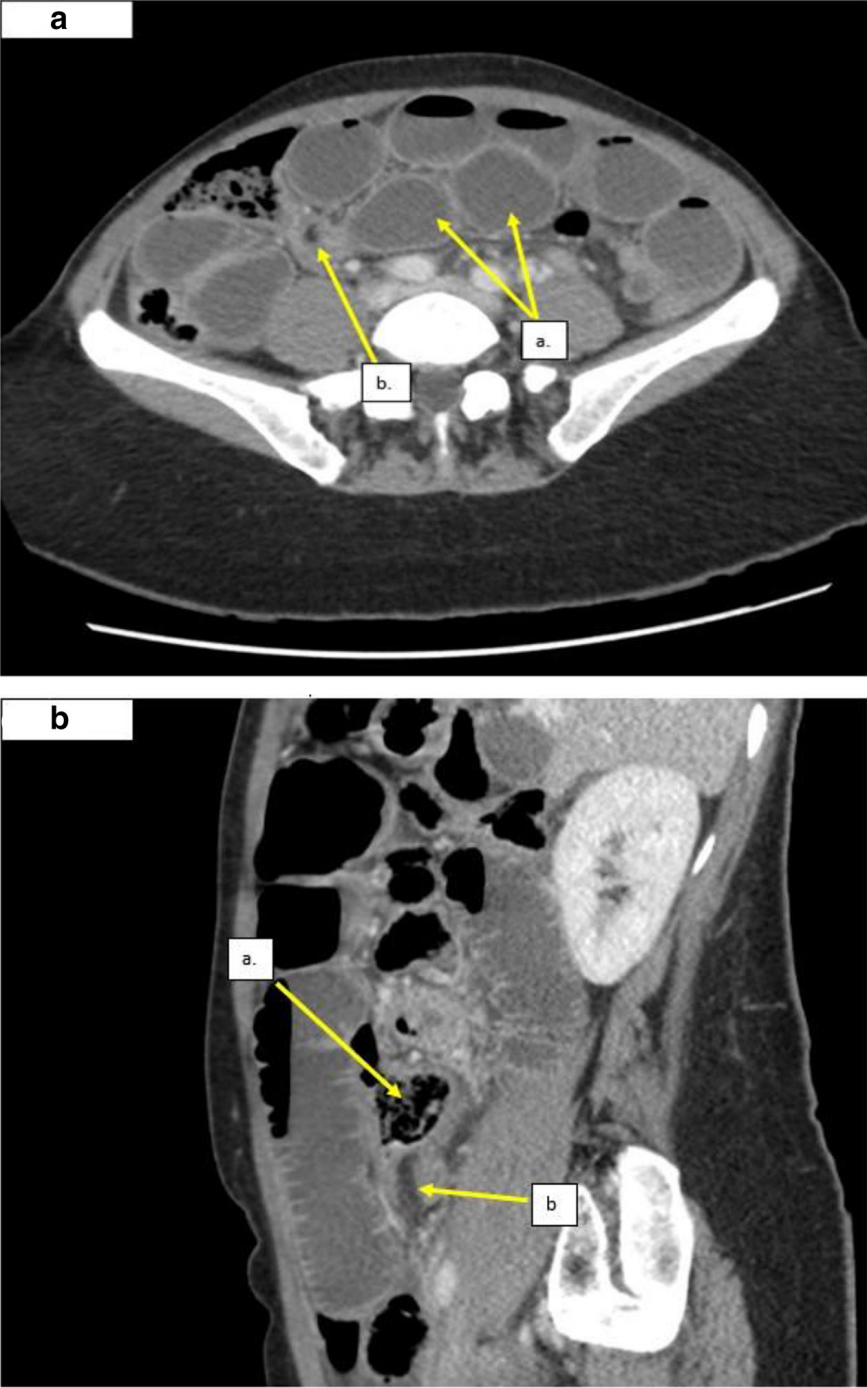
a. Axial CT images showing multiple loops of dilated and fluid-filled small bowel with a linear fat density structure at the transition point (Case 2) a. Dilated, fluid filled small bowel loops. b. Cross-section through the fibrofatty band demonstrating a fat density center. b. Sagittal images from the same CT demonstrating: a. Small bowel feces within the obstructed small bowel up stream of the acute transition point. (b.) Linear fat density band at the acute transition point, the distal small bowel was collapsed downstream to this.
